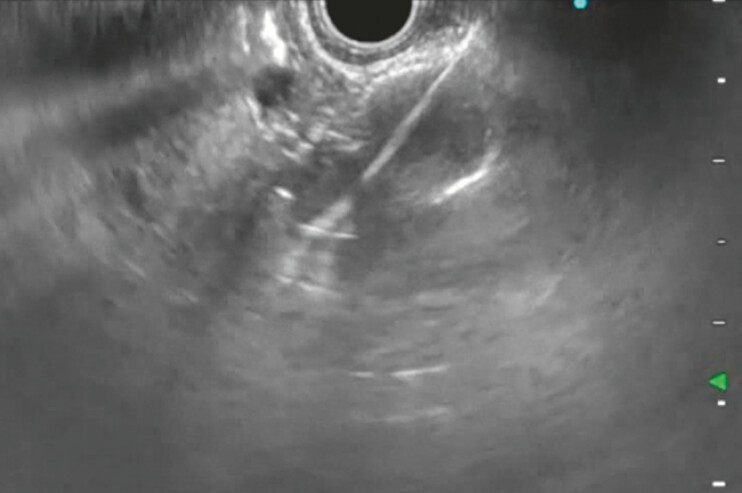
Ultrasound image showing placement of lumen-apposing stent to access the biliary tree in a 65-year-old woman with acute bacterial cholangitis and altered anatomy after previous Roux-en-Y hepaticojejunal anastomosis.
